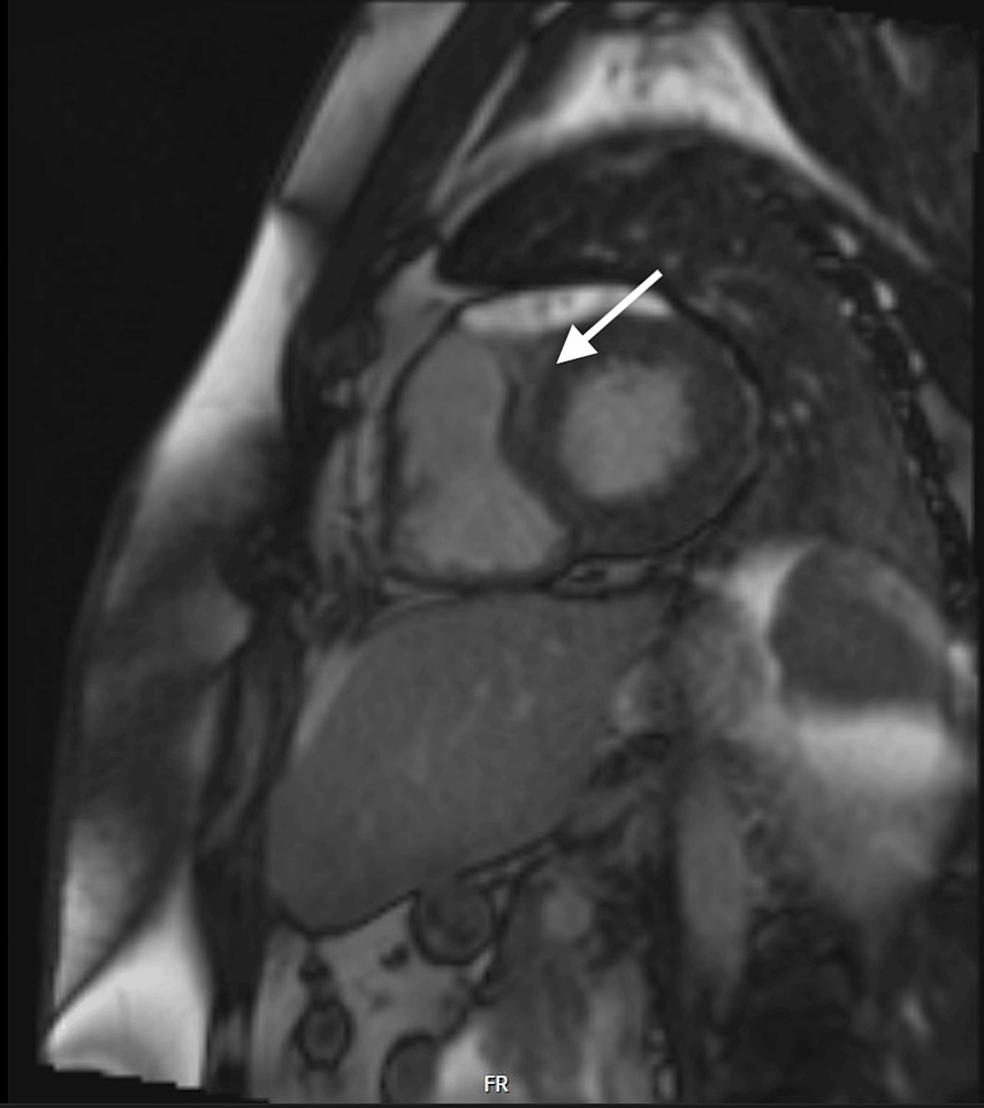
Cardiac MRI Late gadolinium enhancement (LGE) at the basal antero-septal segment (white arrow).
